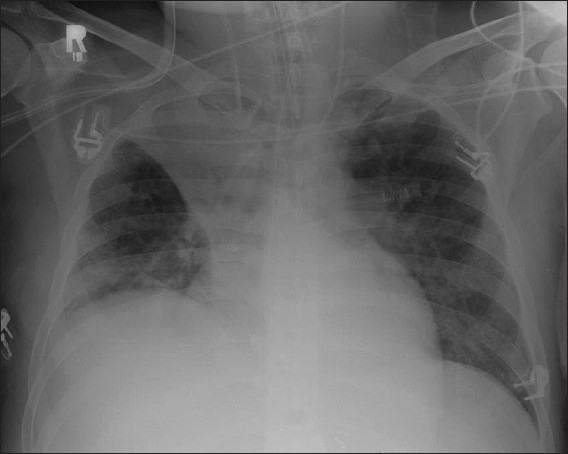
The right upper lobe collapses into a triangular opacity with the lesser fissure migrating toward the anterior, superior and medial portions of the chest, closing like a Chinese fan. On an AP chest radiograph, the most striking feature is a superior and medial displacement of the minor fissure. Note also the raised right hemidiaphragm. On the lateral radiograph (not shown), the major fissure moves anteriorly, while the superior movement of the minor fissure is also seen. This atelectasis was secondary to a mucusplug
